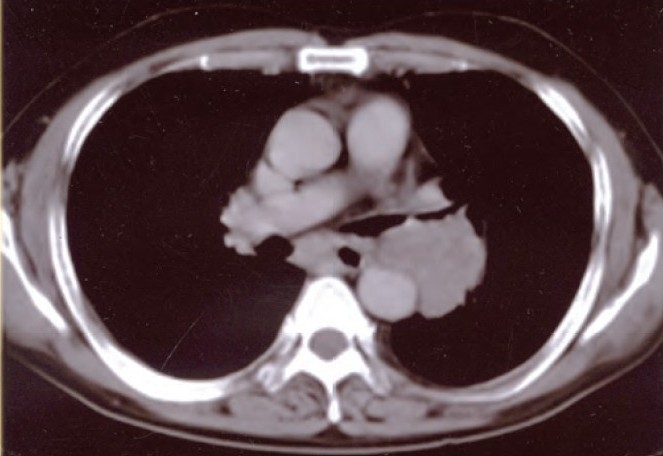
Contrast enhanced computed tomography scan of the chest showing the left hilar mass compressing the left main bronchus with enlarged subcarinal lymph nodes.
